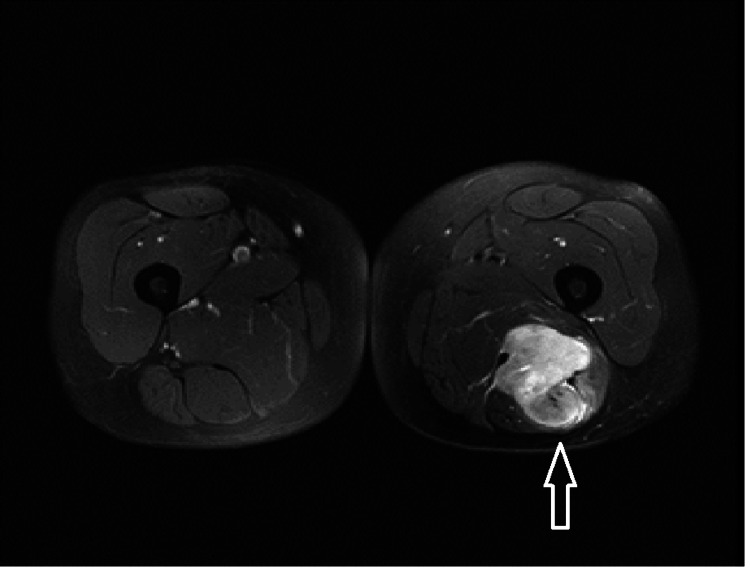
MRI of the thighs showing a heterogeneous tumor (arrow) of the thigh encasing partially the sciatic nerve, measuring 6*6 cm
